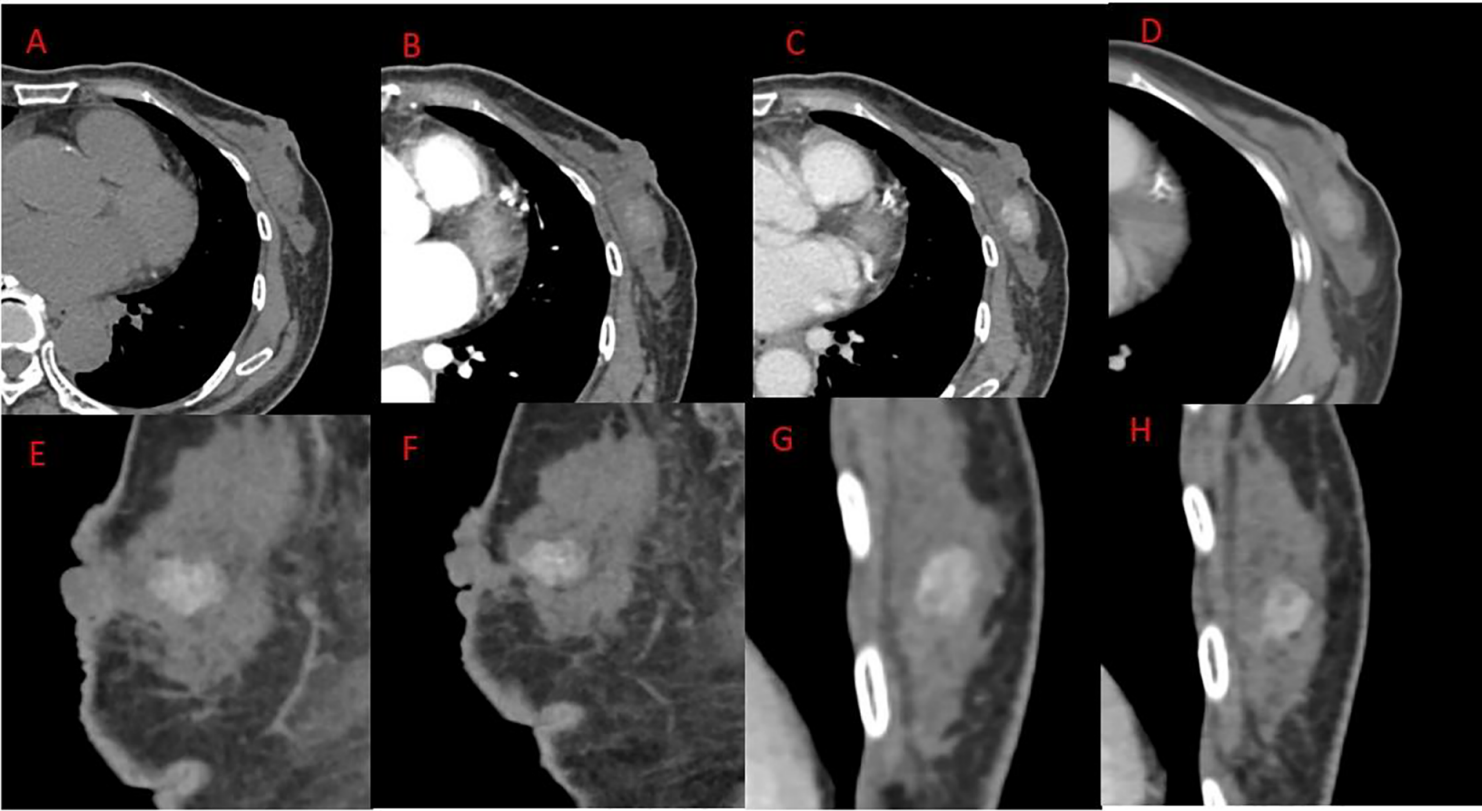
Chest CT plain + enhanced. (A) Plain scan phase, (B) arterial phase, (C) venous phase, (D) delayed phase, (E, F) venous phase sagittal, (G, H) venous phase. Coronal outside left breast first-order density soft tissue nodules were seen in the quadrant, 5 mm distant from the breast. An enhanced scan showed mild and uniform enhancement in the arterial phase and delayed enhancement in the venous and delayed phases. The edge of the lesion was unclear, and no calcification was found within it.
